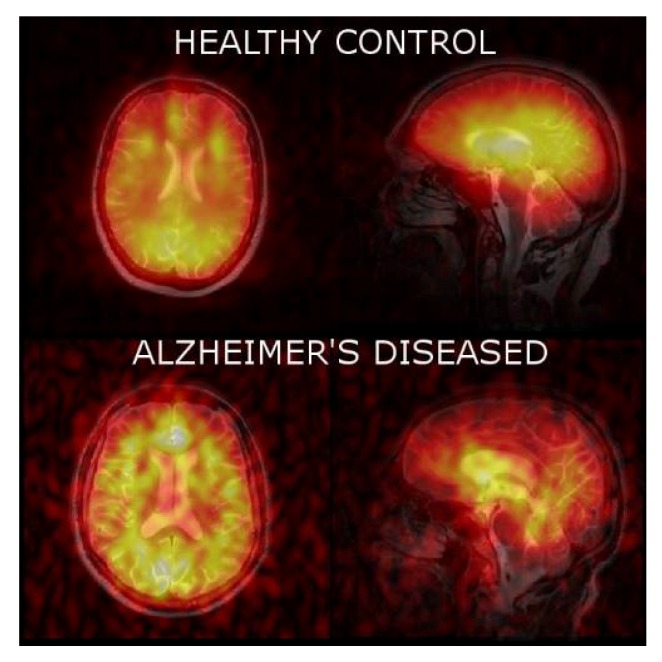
Axial and sagittal ^129^Xe MRI of healthy controls and AD participants. An observably higher SNR was obtained for healthy controls relative to AD participants.
